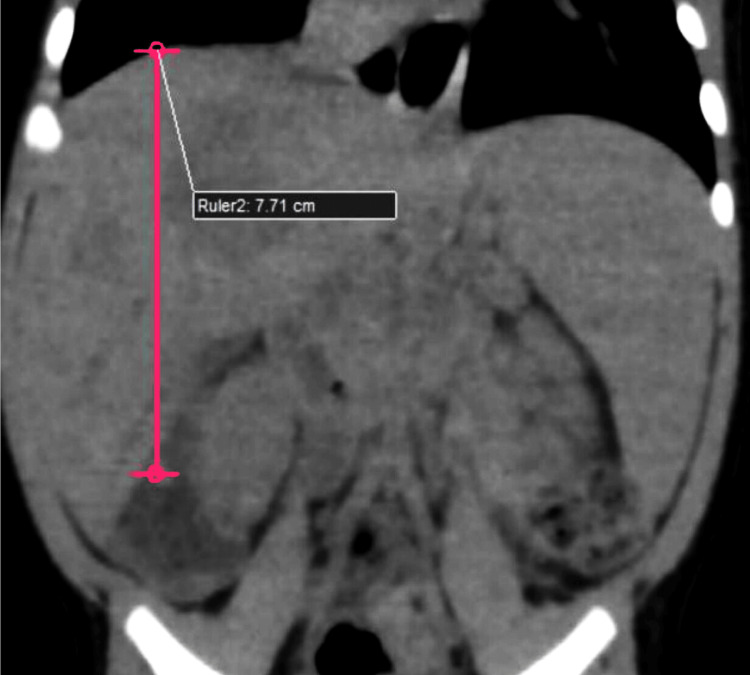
Reformatted coronal section of non-contrast CT image of the abdomen showing hepatomegaly (pink marker) CT: computed tomography
